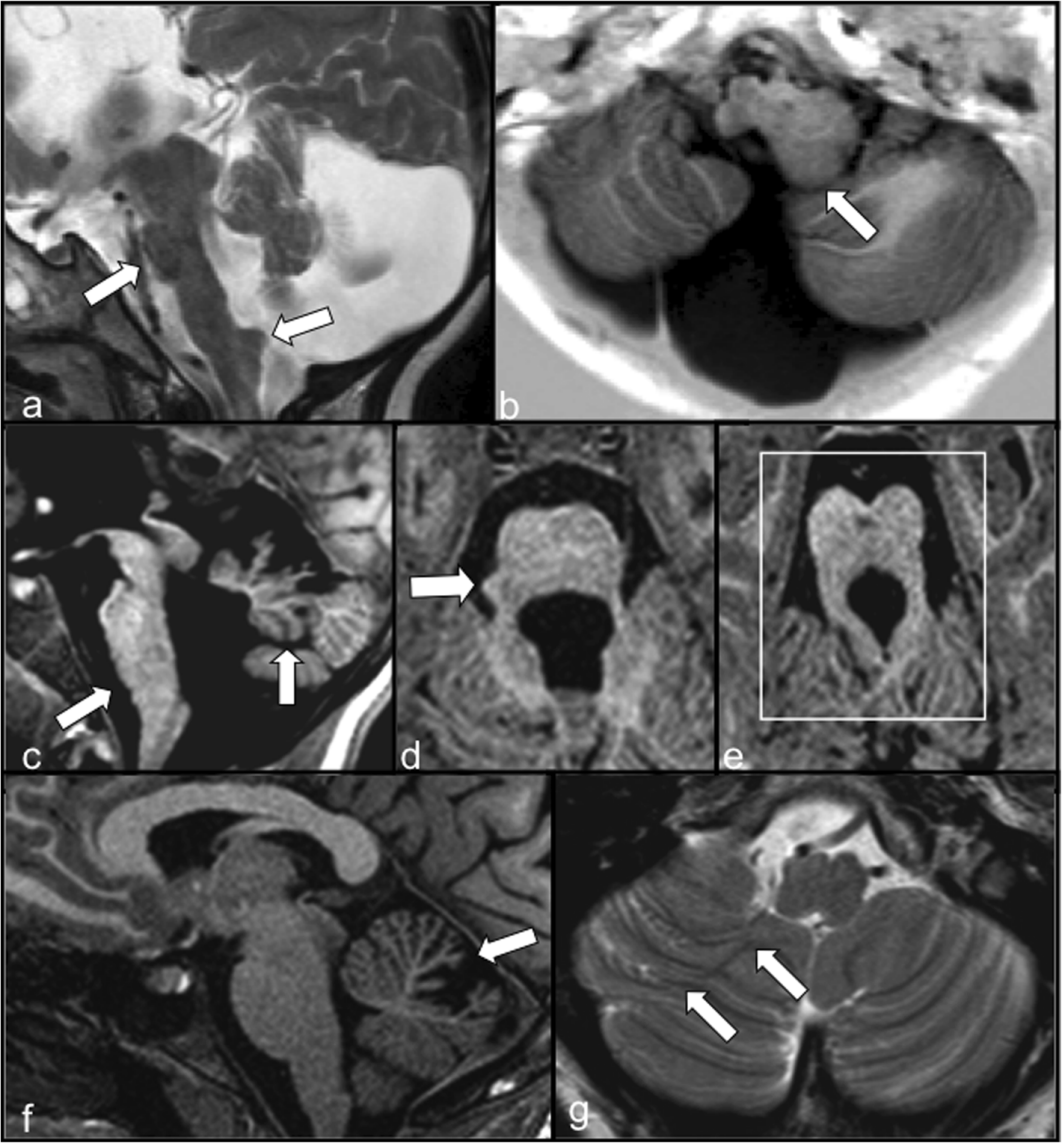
Details of the neuroimaging findings from selected patients. a, b: *Case ID50, 52 months* (*OFD1* mutation: 337 C > T; Q113X)*.*a) Sagittal TSE T2-weighted image: a) vermis and pons hypoplasia (arrow) with anomalous elongation of the medulla, slight swelling of the tectum and aqueductal stenosis are shown together with an enlargement of the fourth ventricle and of the posterior fossa. An anomalous protuberance on the dorsal surface of the medulla is also evident (arrow). b) Axial IR T1-W image: dysmorphism of the left side of the medulla that is bigger than the controlateral one (arrow). Also note the hypoplasia of the cerebellar hemispheres with anomalous orientation of the folia on the right side. c, d, e: *Case ID39, 8-year-old* (*OFD1* mutation: 290 A > G; E97K)*.* c) Sagittal TFE T1weighted 3D image: marked hypogenesis, anticlockwise rotation (arrow) and dysmorphism of the vermis and flat ventral pons (arrow). The fourth ventricle and the posterior fossa are both enlarged. d) Axial TFE T1-weighted 3D image: little protuberance on the right side of the pons (arrow). e) Axial TFE T1 weighted image: typical “molar tooth sign” (square) characterized by thickening and horizontalization of the superior cerebellar peduncles. A mild hypointense dilatation of a Virchow-Robin space is present in the middle of the midbrain. The anomalous orientation of the cerebellar folia is also visible in the superior part of the cerebellum. f, g: *Case ID13, 13-year-old* (*OFD1* mutation: 247CAA > TAA; Q83X)*.*f) Sagittal TFE T1-weighted 3D image: abnormal dilatation of the primary cerebellar fissure and hypoplasia of the declive lobule (arrow). g) Axial TSE T2-weighted image: enlargement of the right biventer lobule with abnormal orientation of the folia (arrows).
